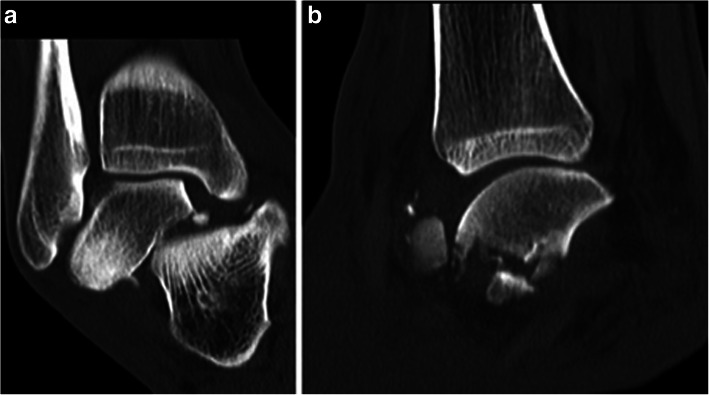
Medial subtalar dislocation associated with talar neck fracture in a 27-year-old man. CT sections (a & b)
